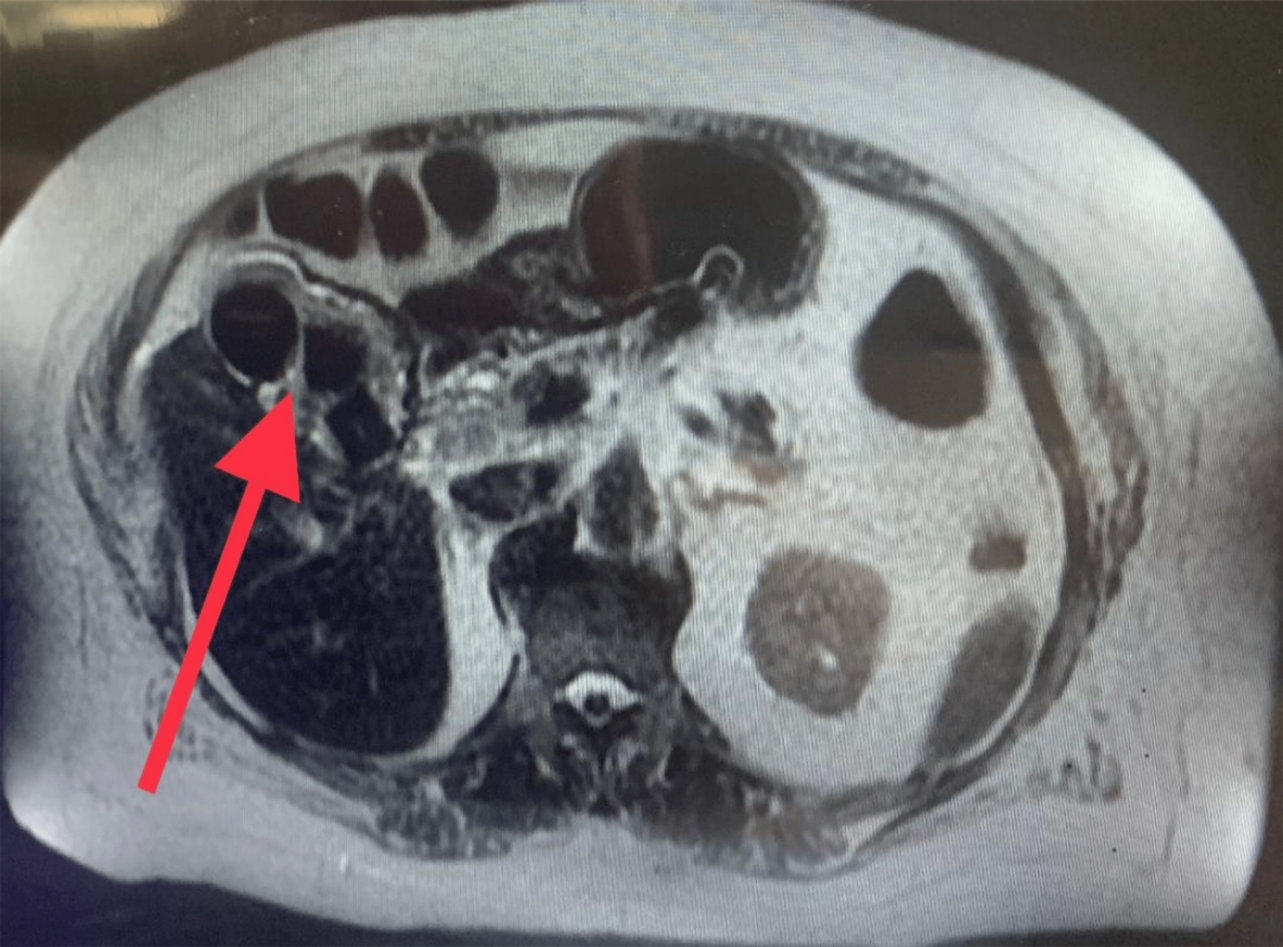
Magnetic resonance imaging of the abdomen. Inflamed gallbladder with large stones (red arrow).
